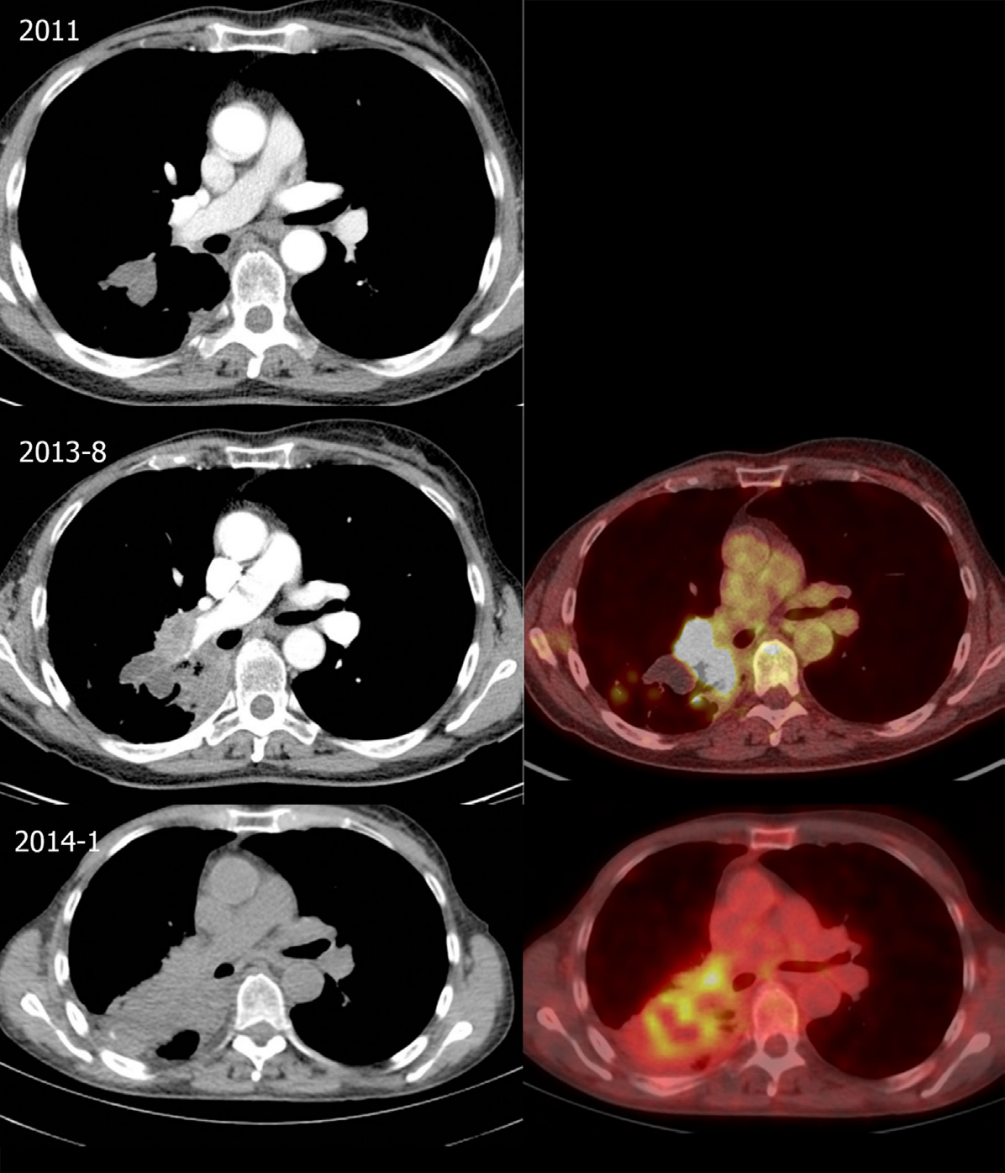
Evolution of thoracic lesions. Top: Follow-up chest computed tomography (CT) in 2011 showing a right-sided lobulated pulmonary mass at the right lower lobe (3.0-cm diameter). Middle: CT (left) and fusion 18-fluordeoxyglucose positron emission tomography-computed tomography (^18^FDG-PET-CT) (right) in August 2013 shows an increase at the medial side of the mass and right hilar lymphadenopathy, with intense 18-fluordeoxyglucose (^18^FDG) uptake. Bottom: CT (left) and fusion ^18^FDG-PET-CT (right) in January 2014 showing further growth of the ^18^FDG-avid mass in the right lower lobe with hilar invasion and a mild ^18^FDG-avid subcarinal lymph node.
